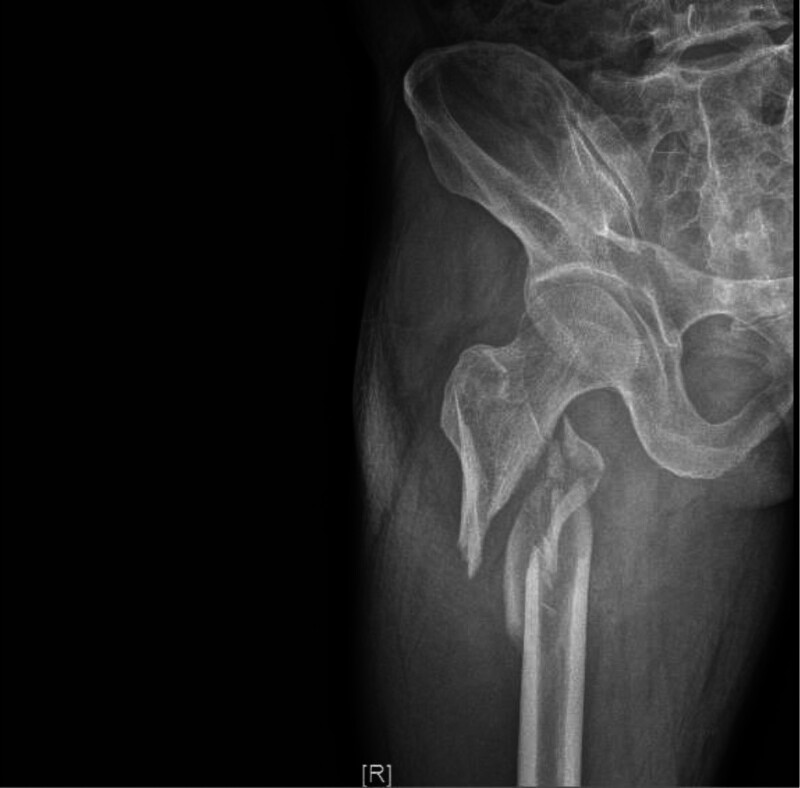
The X-ray after the injury shows a fracture below the femoral trochanter.
